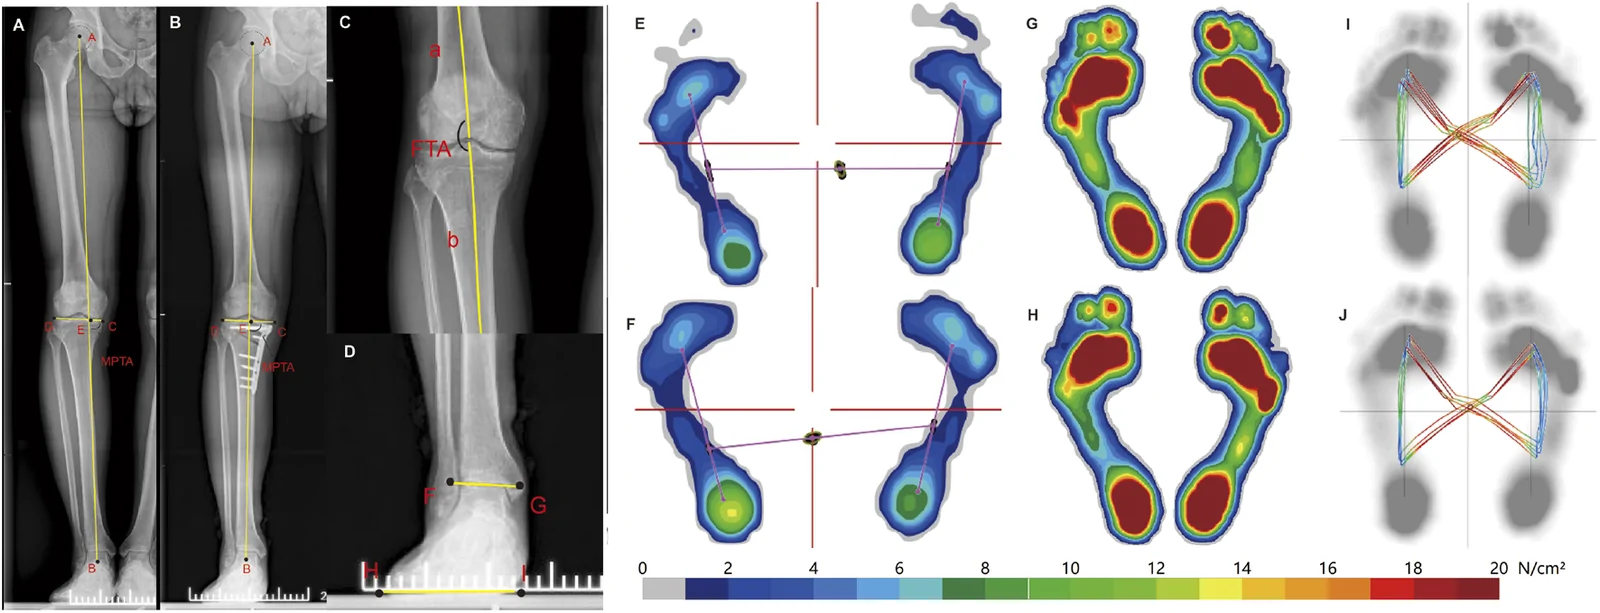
Radiographic, plantar-pressure, and gait evaluation of a representative patient pre- and postoperatively (A,B) Preoperative and postoperative full-length standing anteroposterior radiographs of the same patient. The medial proximal tibial angle (MPTA) is defined as the medial angle (∠CEB) formed between the tibial mechanical axis (line AB) and the tangent to the tibial plateau (line CD). The weight-bearing line ratio (WBLR) represents the percentage of the tibial plateau width traversed by the mechanical axis, calculated as CE/CD × 100%, where C is the intersection point of the mechanical axis with the tibial plateau and E is the medial edge of the plateau (C) The femorotibial angle (FTA) is the angle between the anatomical axis of the femur (line a) and that of the tibia (line b) (D) The tibial plafond–plantar angle (TPI) is the angle between the tangent to the distal tibial articular surface (line FG) and the plantar horizontal line (line HI) (E,F) Center of pressure (COP) alignment: the overall COP deviates from the midline preoperatively (E) and centralizes geometrically postoperatively (F) (G,H) Plantar-pressure thermogram: postoperatively (H), maximum force increases in the left heel, with increased medial force concentration at the first metatarsal (M1) region compared to the preoperative (G) baseline (I,J) Gait butterfly parameters: the preoperative (I) left-shifted COP trajectory centralizes postoperatively (J), demonstrating restored bilateral symmetry.
